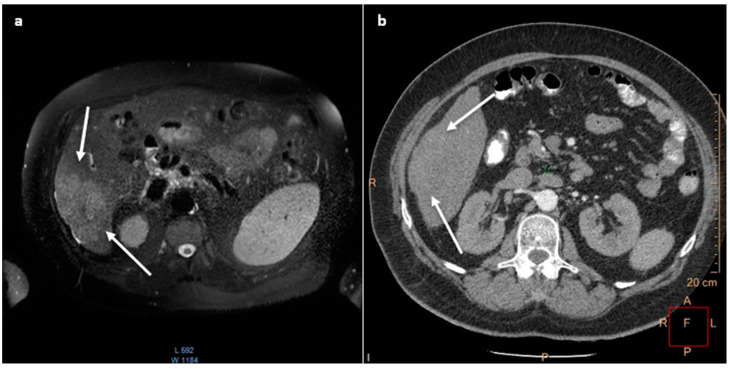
Axial magnetic resonance imaging (a) and computed tomography scan (b) of the abdomen visualizing a hypovascular tumor of the right lobe of the liver (white arrows) with no arterial enhancement.
